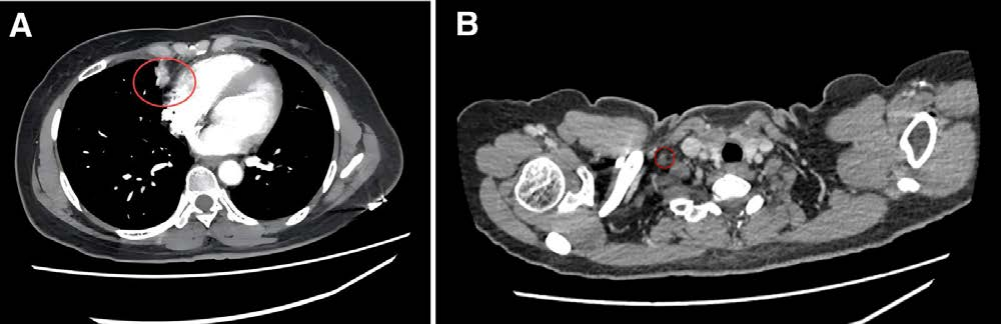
Chest CT follow-up on January 20, 2021. (A) Continued reduction in the right lung lesion; (B) continued reduction in the right supraclavicular lymph node. CT = computed tomography.
